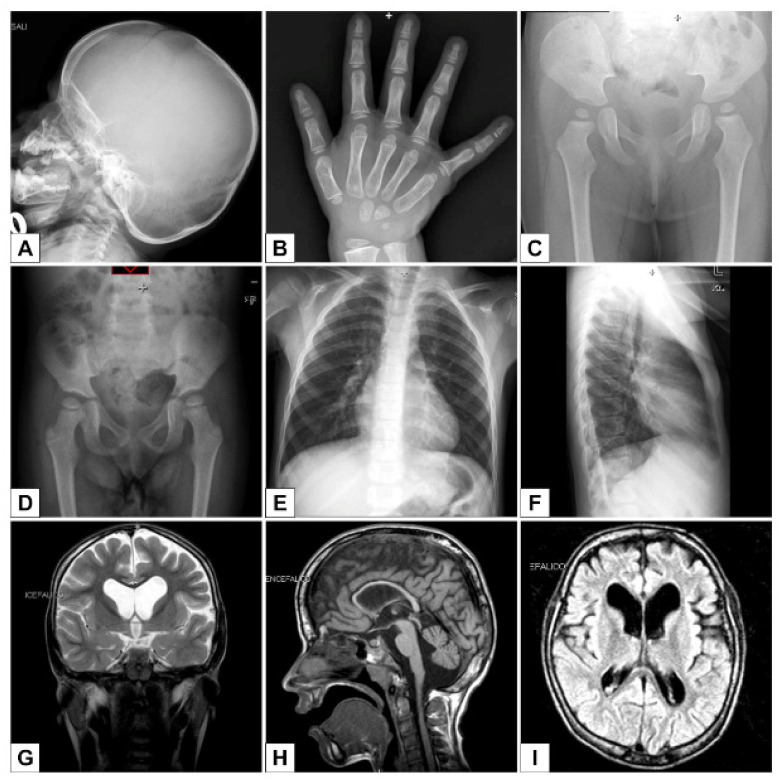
(A) Skull X-ray: large fontanel and Wormian bones; (B) Hand X-ray: delayed bone age. According to Greulich and Pyle Atlas at a chronological age of 4 years and 9 months, her bone age was consistent with an age of 2 years and 5 months; (C,D) Hip X-rays at 1 and 6 years: hip dysplasia; (E,F) Spine X-rays: scoliosis and mild thoracic kyphosis; (G–I) Brain MRI; (G) Coronal DWI image: large frontal horns of the lateral ventricles; (H) Sagittal T1 image: cerebellar atrophy and thin corpus callosum; (I) Axial FLAIR image: frontal lobes atrophy and large frontal horns of the lateral ventricles.
